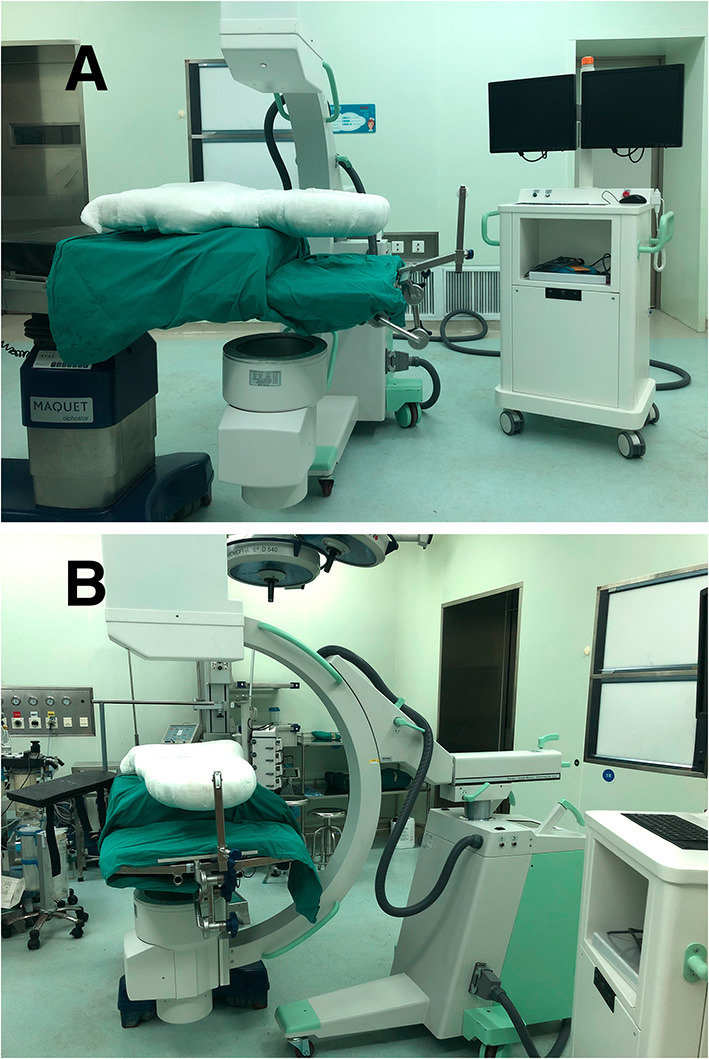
The BSPST system and C-arm digital radiography machine from different positions (A,B), which can make anterior-posterior interoperative radiography available.
